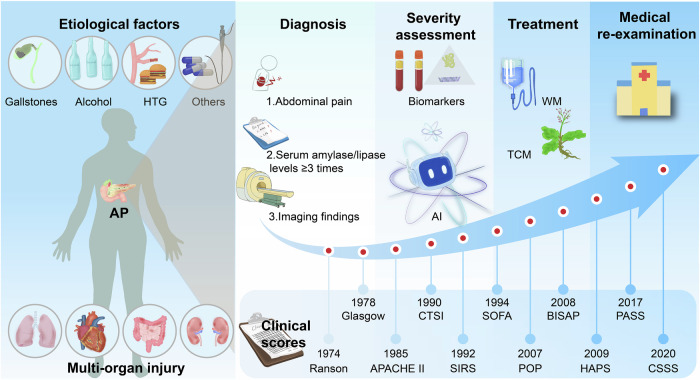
Overview of acute pancreatitis. This figure summarizes the current understanding of epidemiology, diagnosis, severity assessment, and treatment approaches. AI artificial intelligence, AP acute pancreatitis, APACHE II acute physiology and chronic health evaluation II, BISAP bedside index of severity in acute pancreatitis, CSSS Chinese simple scoring score, CTSI computed tomography severity index, HAPS harmless acute pancreatitis score, HTG hypertriglyceridemia, PASS pancreatitis activity scoring system, POP pancreatitis outcome prediction score, SIRS systemic inflammatory response syndrome, SOFA sequential organ failure assessment, TCM traditional Chinese medicine, WM western medicine
